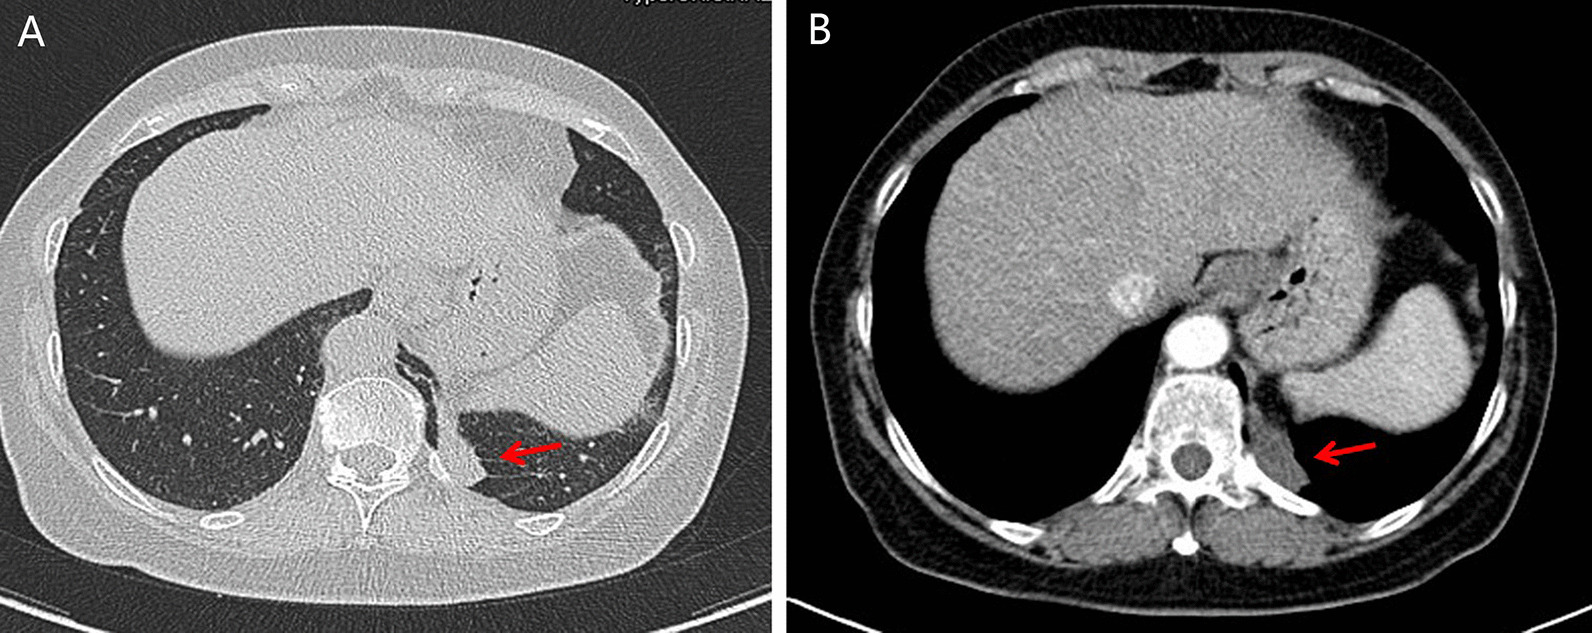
A, B Computed tomography (CT) of chest with contrast showed a 6 × 5 cm soft tissue in the posterior basal segment of the left lower lobe of the lung, and its adjacent pleura is thickened (arrows)
